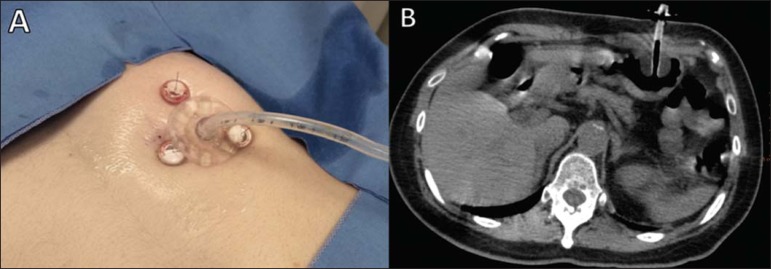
Final step of CT-guided percutaneous gastrostomy. After the correct
